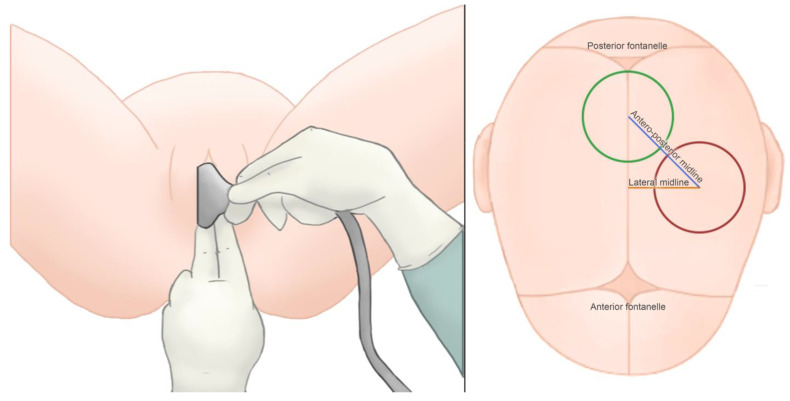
(Left) Ultrasound assistance for vacuum cup placement, with probe placed in the sagittal transperineal plane. (Right) Measurement of the distance between the chignon (red) and flexion point (green).
